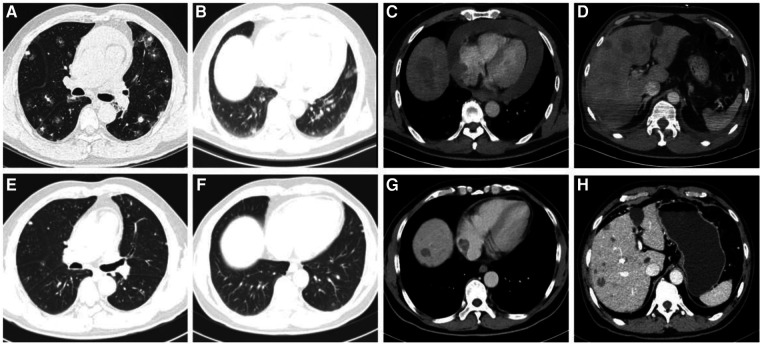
(A–D) Chest CT scan of the patient revealed dispersed sub-solid ground glass shadows in both lungs, soft tissue shadows in the right atrium, numerous nodular shadows in the liver, and a considerable pericardial effusion before treatment. (E,F) After 4 cycles of anlotinib treatment, the patient's numerous nodules in the lungs and liver showed significant reduction, the tumor lesion in the right atrium decreased, and pericardial effusion as managed.
